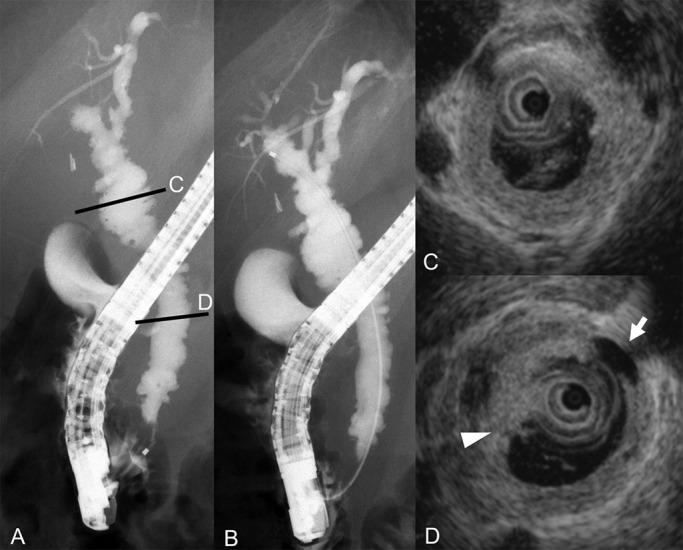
Findings of endoscopic retrograde cholangiography and intraductal ultrasonography on admission to our hospital. A–B) Endoscopic retrograde cholangiography revealed diverticulum-like outpouching of the distal common bile duct and a pruned-tree appearance of intrahepatic bile ducts. C–D) Intraductal ultrasonography demonstrated sludge (arrowhead) and diverticulum-like outpouching (arrow).
